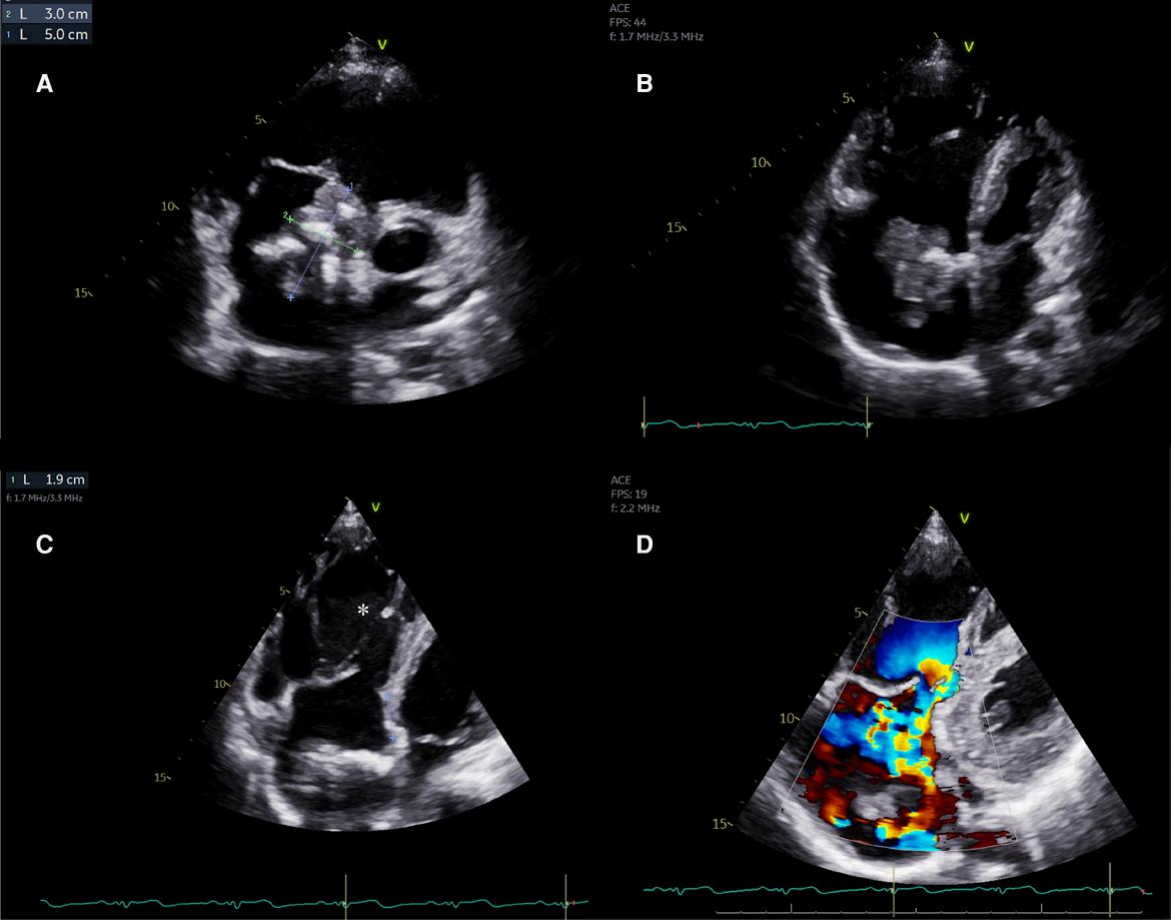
Echocardiogram showing (*A*) irregular and heterogeneous mass in an enlarged right atrium, (*B*) significant right heart dilatation, (*C*) apical displacement of the septal leaflet of the tricuspid valve (*), and (*D*) severe tricuspid regurgitation.
